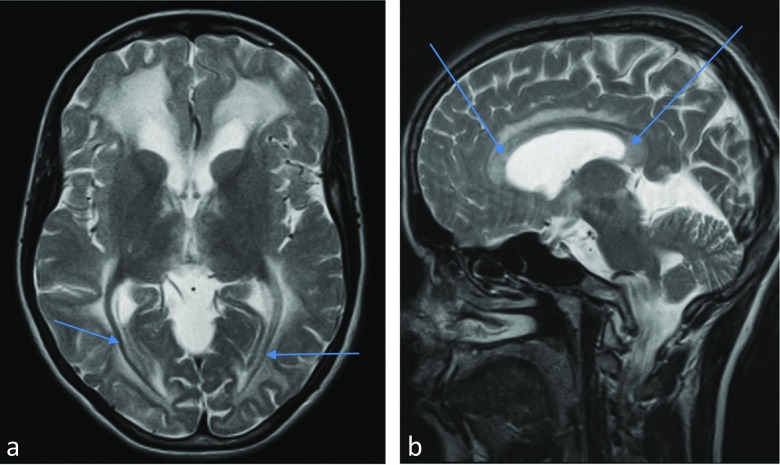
MRI - a Bilaterally preserved myelination of the optic radiation; b Abnormal signal of the corpus callosum
